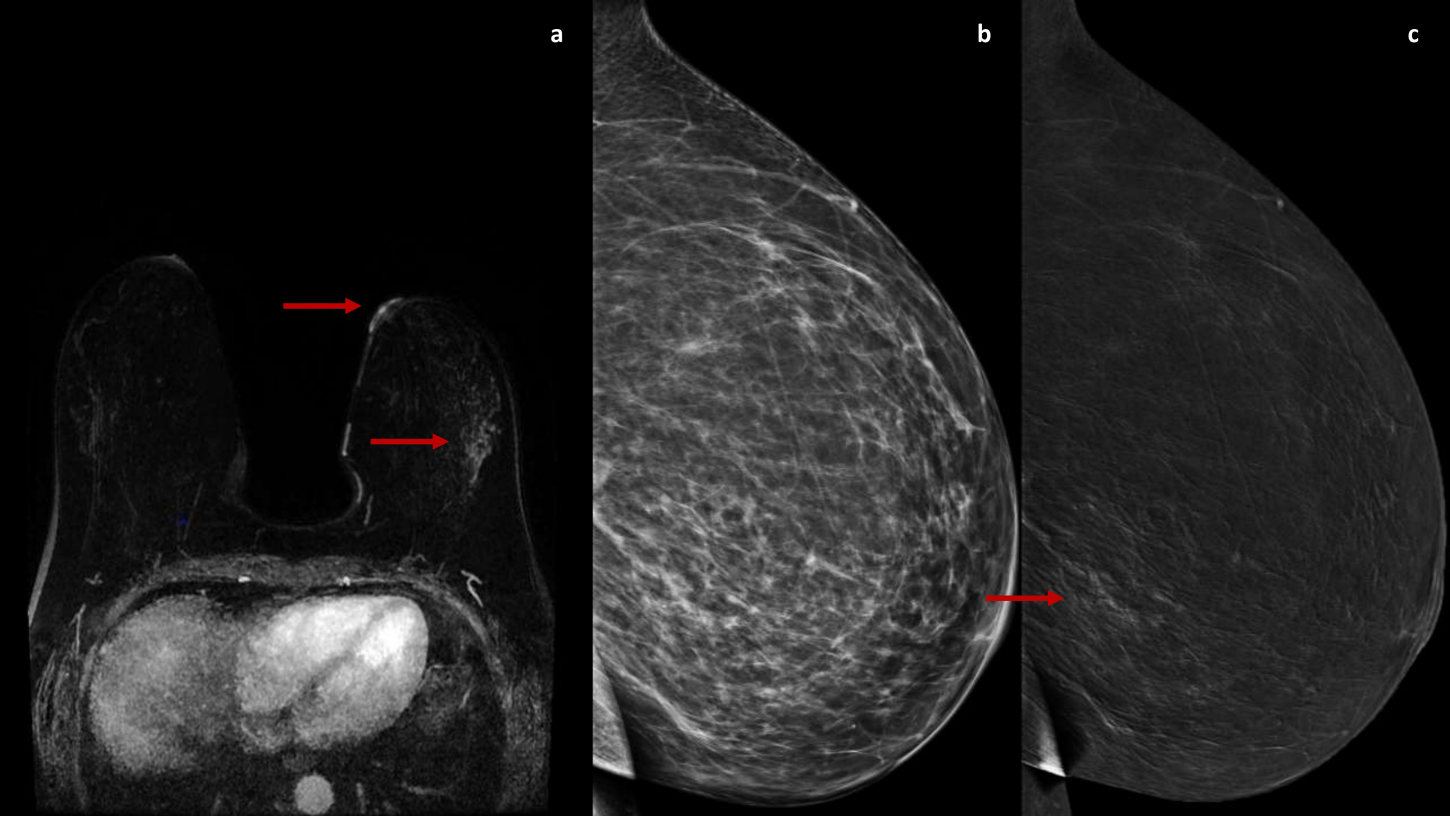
A DCE-MRI subtraction image (A) shows an enhancement of the left nipple with a non-mass-like enhancement in the outer quadrants (arrow). The 2D and recombined medio-lateral CEM images (B, C) confirm a later inhomogeneous non-mass-like enhancement in the outer quadrants of the left breast (arrow).
